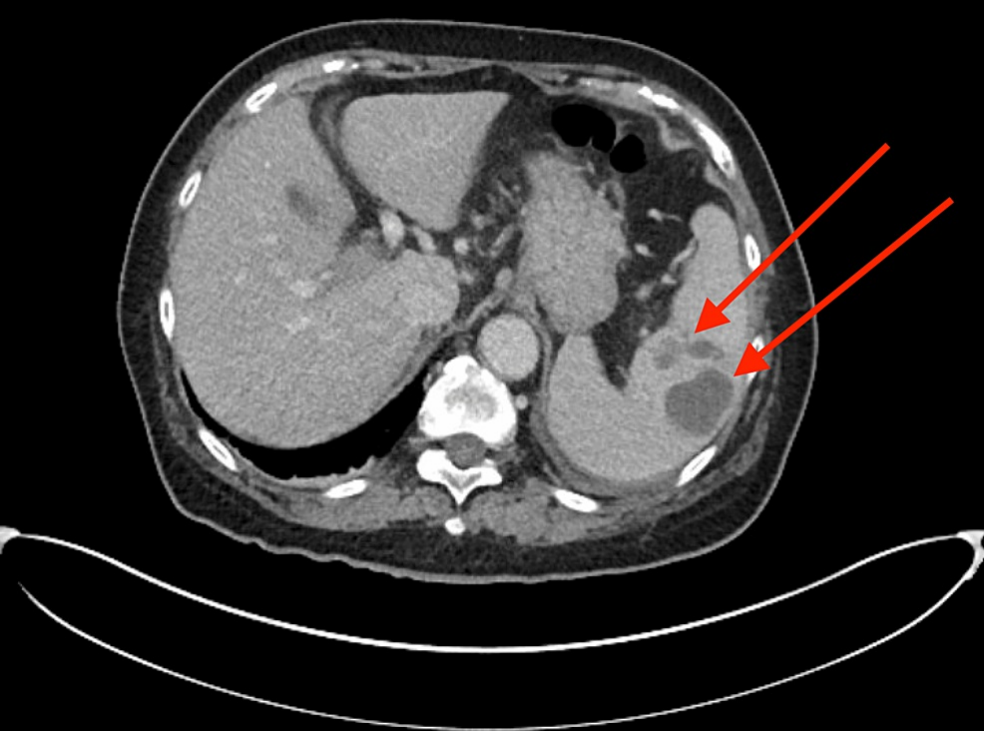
Axial CT view of multiple hypoenhancing lesions (red arrows) consistent with splenic abscesses on the patient’s admission.
